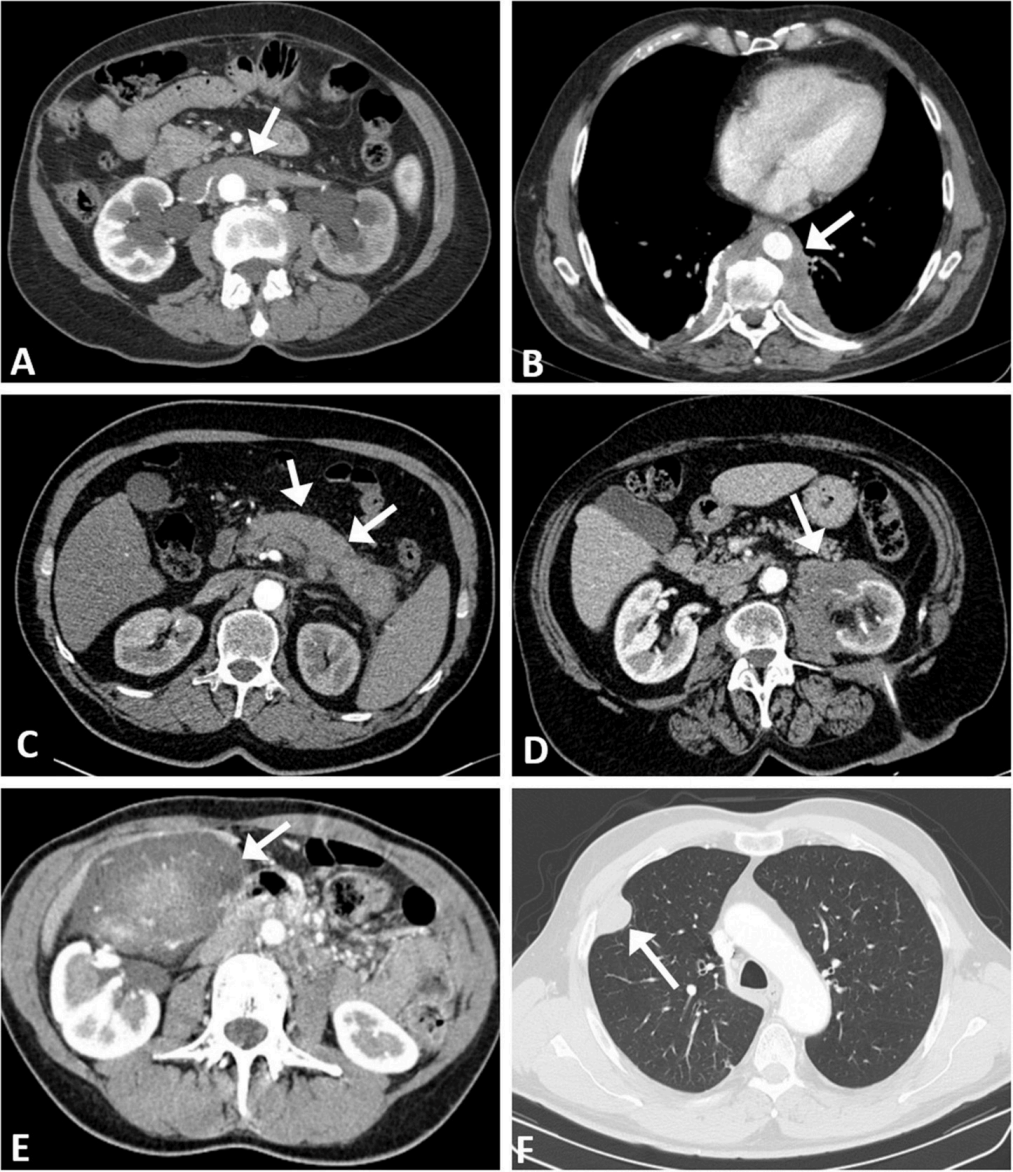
CT Imaging overview of chronic periaortitis and extra-retroperitoneal lesions. (A) Sleeve thickening of the abdominal aorta (*arrow*) with involvement of the renal arteries; the fibro-inflammatory tissue wraps around the excretory system and determines bilateral hydronephrosis. (B) Typical aspect of “coated aorta” involvement along the descending thoracic tract: the inflammatory tissue (*arrow*) also expands into the paravertebral space and envelops the verterbal bodies. (C) IgG4-related chronic pancreatitis: the normal glandular structure is subverted (*arrows*) and a pseudonodular lesion is evident at the level of the pancreatic tail. (D) Atypical localization of retroperitoneal fibrosis: the pathological tissue encroaches into the left perirenal space (*arrow*) without involvement of excretory system. (E) Unusual gallbladder localization of IgG4-related disease: a bulky mass (*arrow*) attached to the bottom of the gallbladder with large cholecystic feeding vessels. (F) Thoracic localization: a pseudonodular lesion arises from the right pleural sheet (*arrow*).
